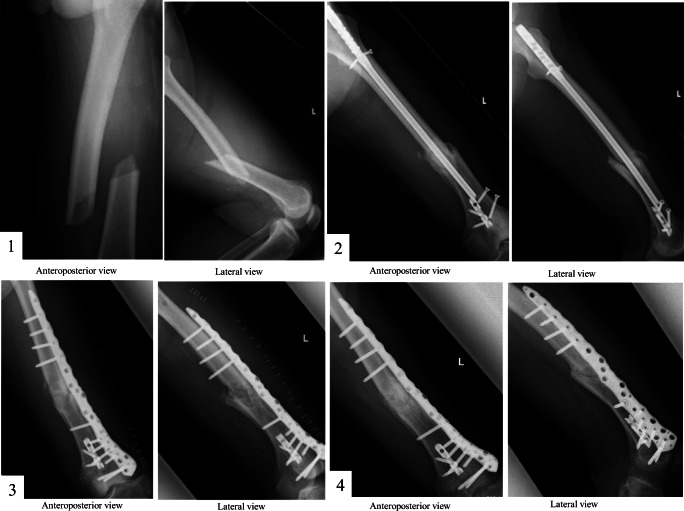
Frontal and lateral radiograph of the left femur. (1), left femoral shaft transverse fracture; (2), bone fixation failure with pseudarthrosis; (3), open reduction and fixation performed using an angular stability plate associated with autogenous bone graft from the patient’s left iliac bone; (4), successful bone union
